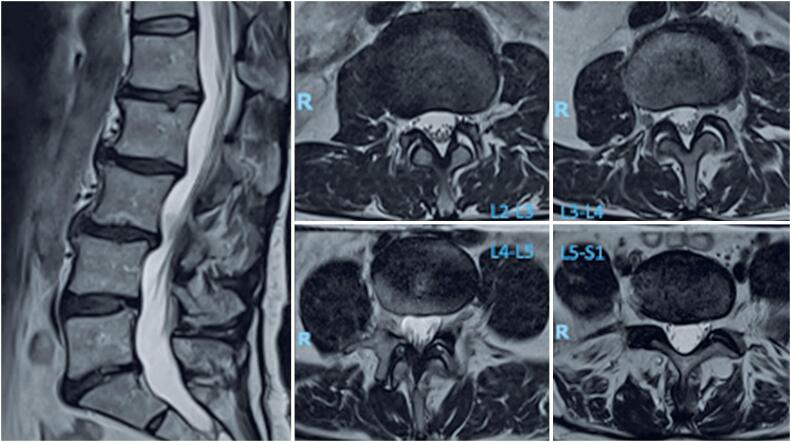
Sagittal central cut and axial cuts by level, T2-weighted magnetic resonance imaging pre-procedure
